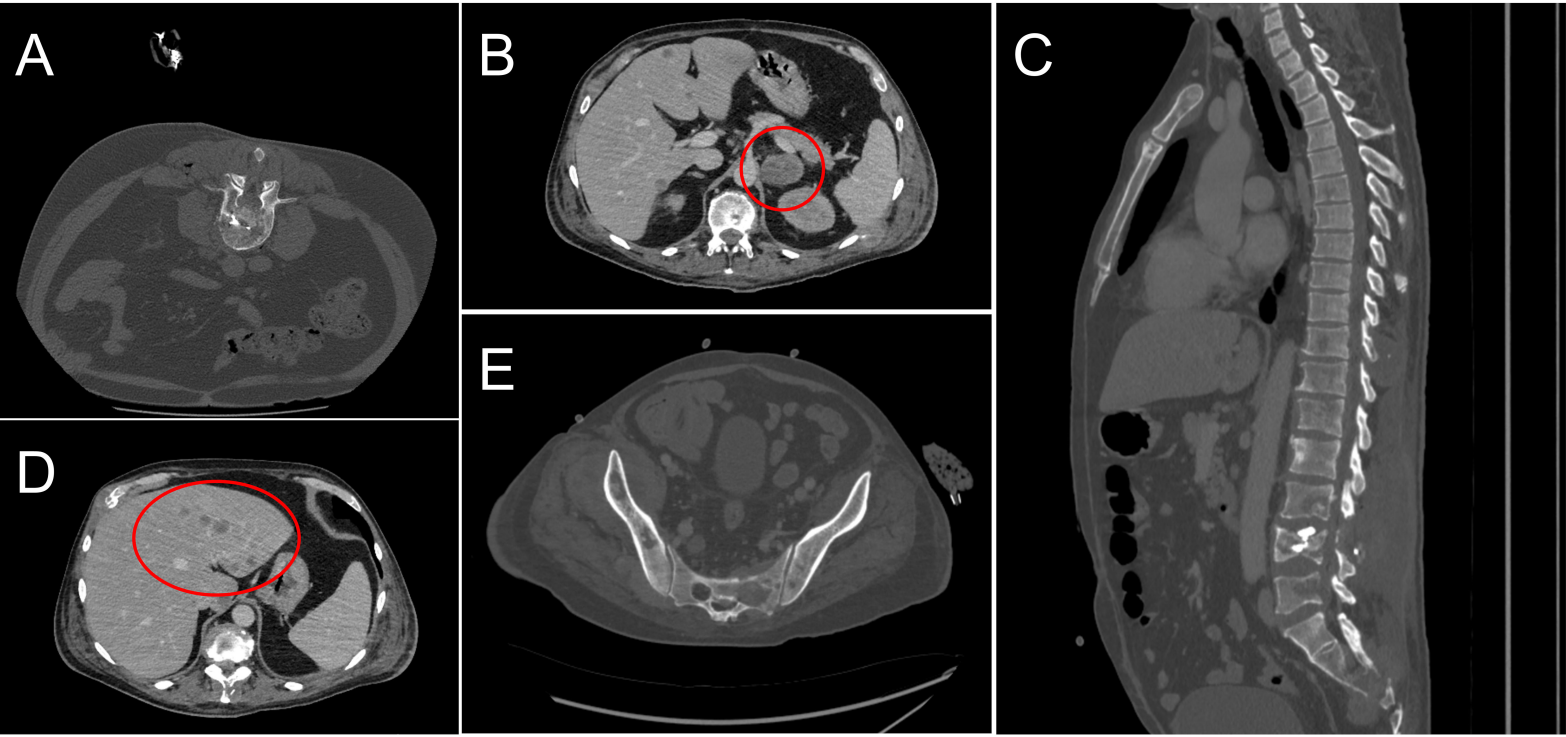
Computed tomography scans of the patient’s metastatic sites in the course of the treatment. (A) Metastasis and pathologic fracture in L4 revealed in June 2023; (B) Metastatic lesions in the adrenal glands, August 2023; (C) Metastatic lesions in the Th11-S5 vertebrae, June 2023; (D) Metastatic lesions in the liver, August 2023; (E) Destruction of pelvic bones, August 2023.
